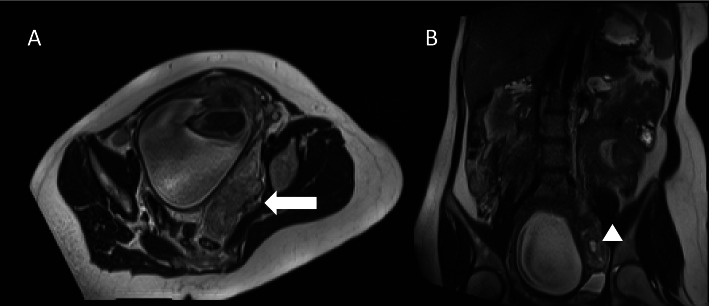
Non contrast-enhanced MRI examination at 1.5 T of the pregnant patient in supine position. A transversal T2w HASTE sequence; B coronal T2w HASTE sequence. Segmental sigmoid involvement of diverticulitis presenting with diffuse wall thickening (A white arrow) and extensive inflammatory changes including a small abscess formation (B white arrowhead) and perisigmoid inflammation
